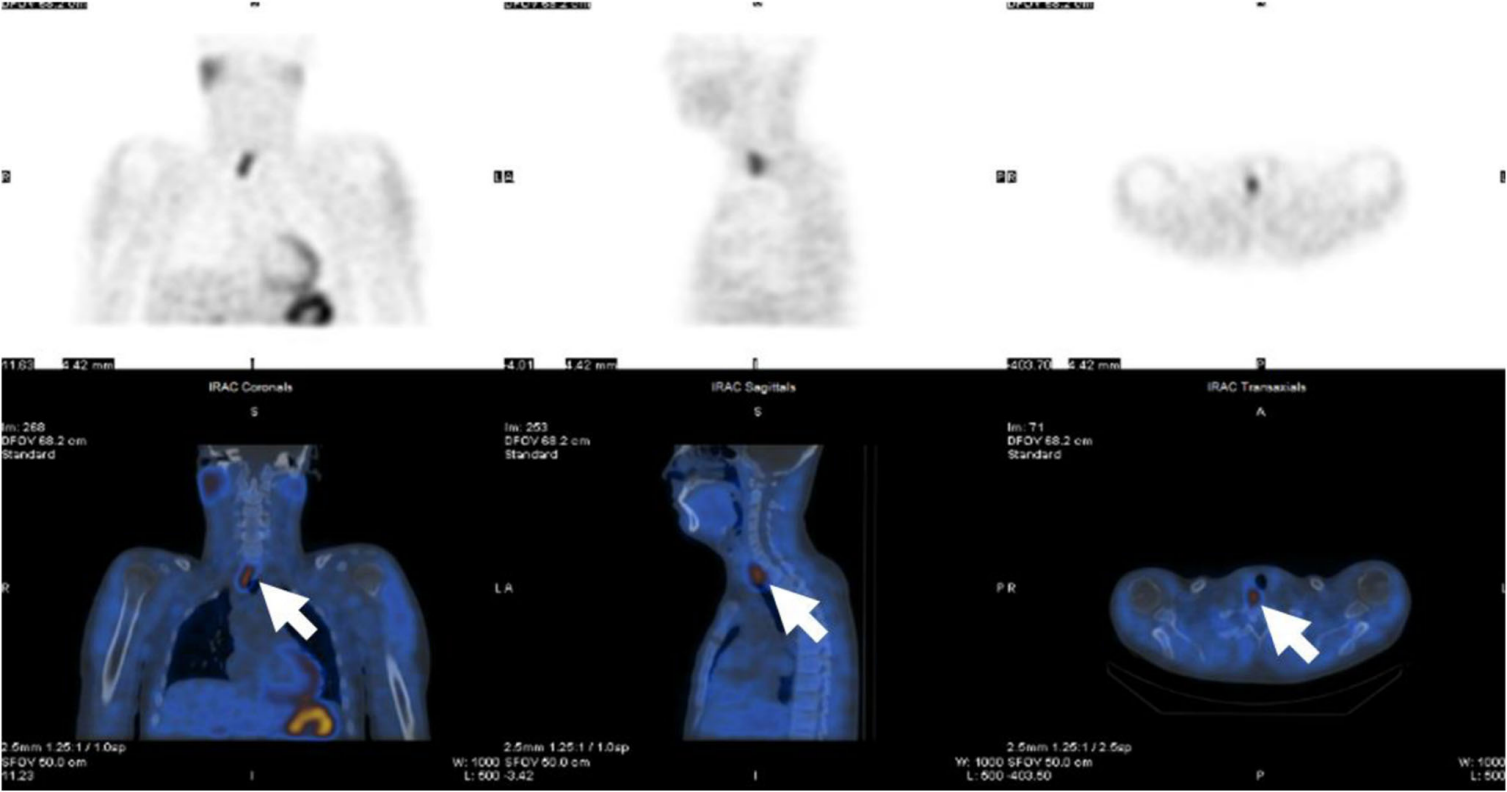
Anterior maximum intensity projection images of SPECT showing delayed washout of radiotracer (upper panel) and delayed-phase coronal, sagittal and axial fused ^99m^Tc-Sestambi-SPECT/CT images showing a parathyroid adenoma localising posterior and inferior to the right lobe of thyroid (lower panel) of a man (case 5)
